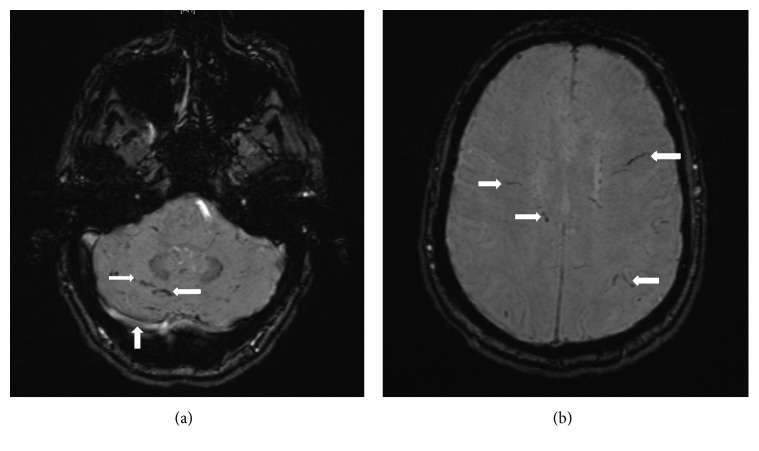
Magnetic resonance imaging of the brain—susceptibility-weighted imaging maximum intensity projection (SWI mip) sequence showing linear and punctate haemorrhagic lesions throughout the cerebrum and cerebellum and leptomeningeal enhancement in the right cerebellum folia.
